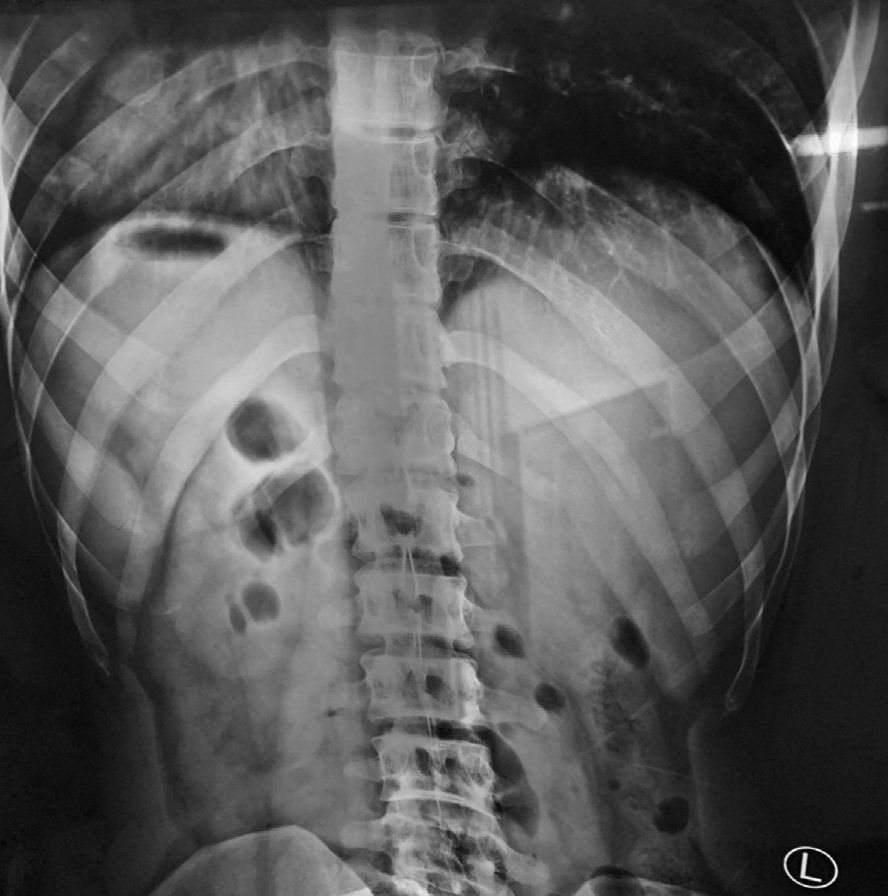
Plain X-ray of the chest and the abdomen showing the cardiac shadow in the right side of the chest and the gastric bubble under the right dome of diaphragm.
